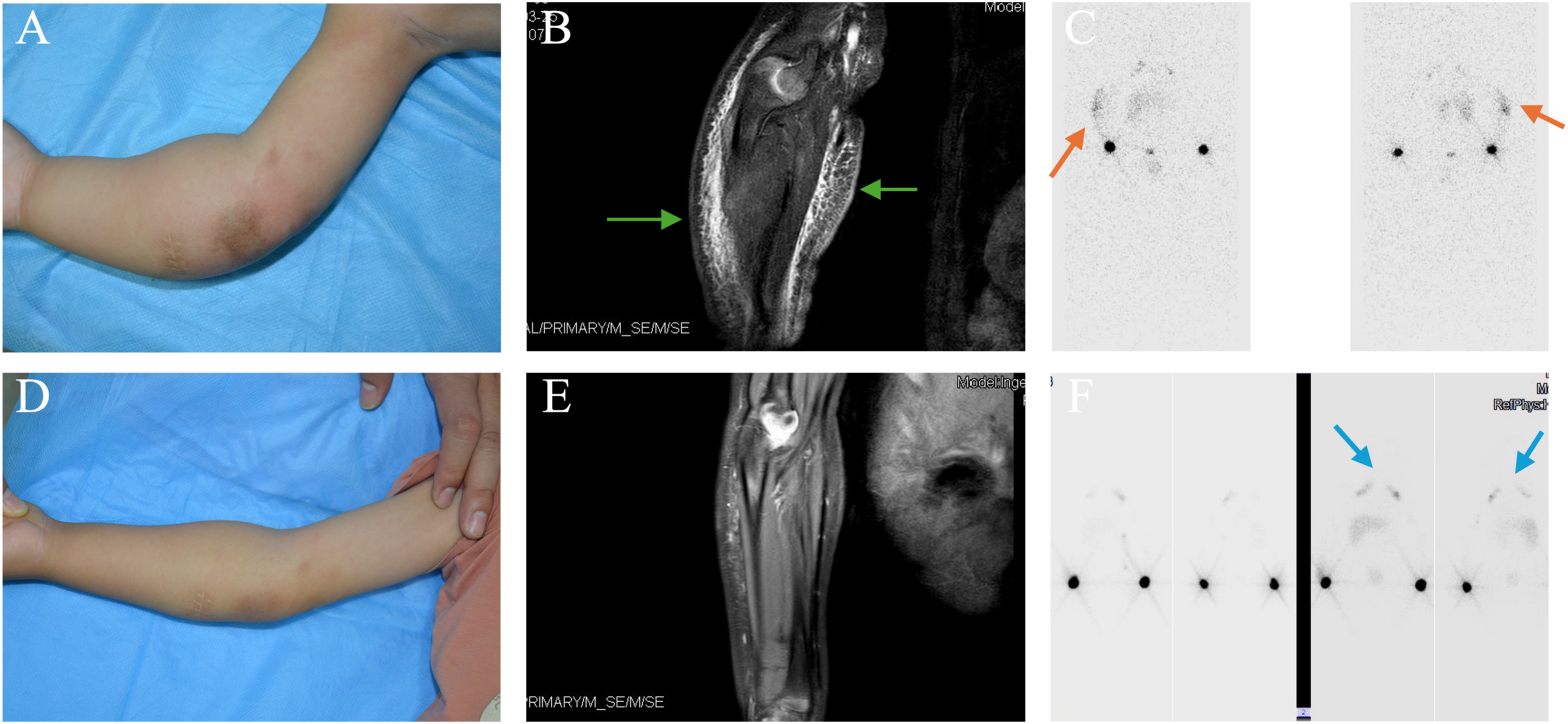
KHE-associated lymphedema affected the right upper extremity in a male patient. Vascular lesions were observed in this patient at birth. Limb swelling occurred 19 months later. (A) The photograph depicts tight swelling on the right arm at referral (at 19 months of age). (B) Coronal T2-weighted MR image revealing hyperintense lesions affecting the dermis, subcutaneous tissue, and deep fascia of the right arm, accompanied by swelling of the surrounding soft tissue (appearing as a blurry dark area, as shown by the green arrows). (C) The nuclear lymphoscintigram acquired 100 and 150 min after injection into the right arm revealed tracer uptake in the left axillary lymph nodes but not on the right side. Notably, abnormal tracer accumulation was observed in the right elbow (orange arrows). (D,E) Fifteen months after compression and medication, the swelling of his right elbow was reduced, and MRI revealed fewer high-intensity lesions in the dermis, subcutaneous tissue, and deep fascia than in panel B. (F) At 34 months of age, lymphoscintigraphy revealed visualization of both sides of the axillary nodes 1 h after injection into the right limb (blue arrows), indicating normal lymphatic reflux of the right upper arm.
